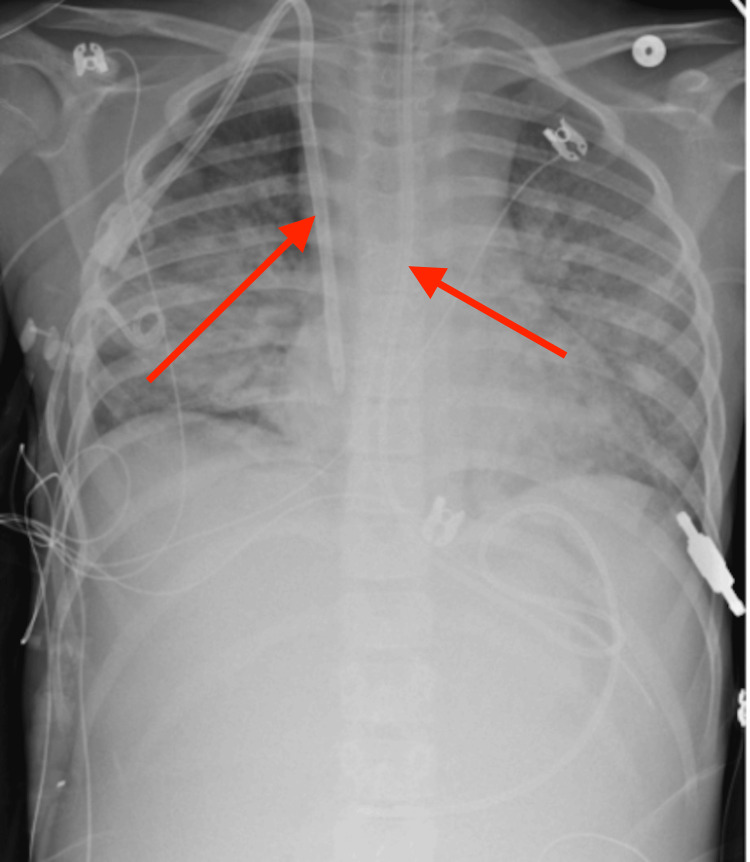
Portable supine anteroposterior chest radiograph This image demonstrates diffuse bilateral patchy airspace opacities with support devices in place, including an endotracheal tube and central venous catheter (red arrows).
